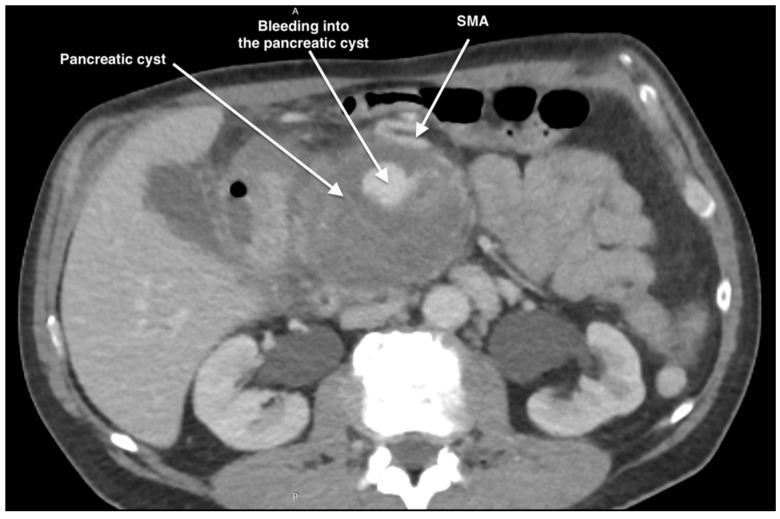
Preoperative CTA aspect of the ruptured SMAA-coronal view describing the course of the SMA and its rupture into the pancreatic cyst.
